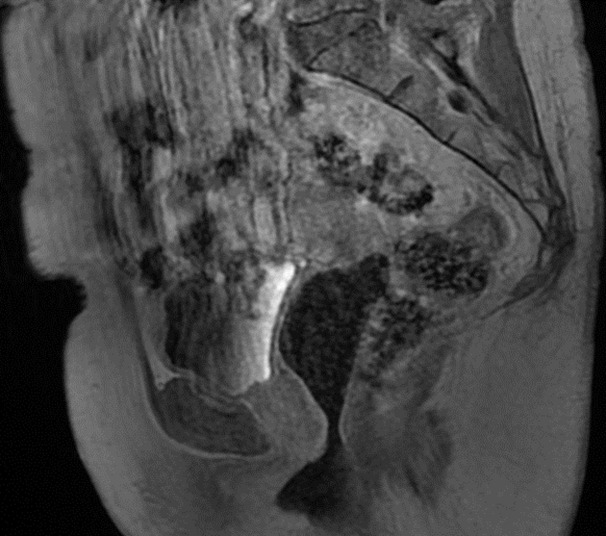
sagittal section of a pelvic magnetic resonance imaging without complications related to the urethral diverticulum
